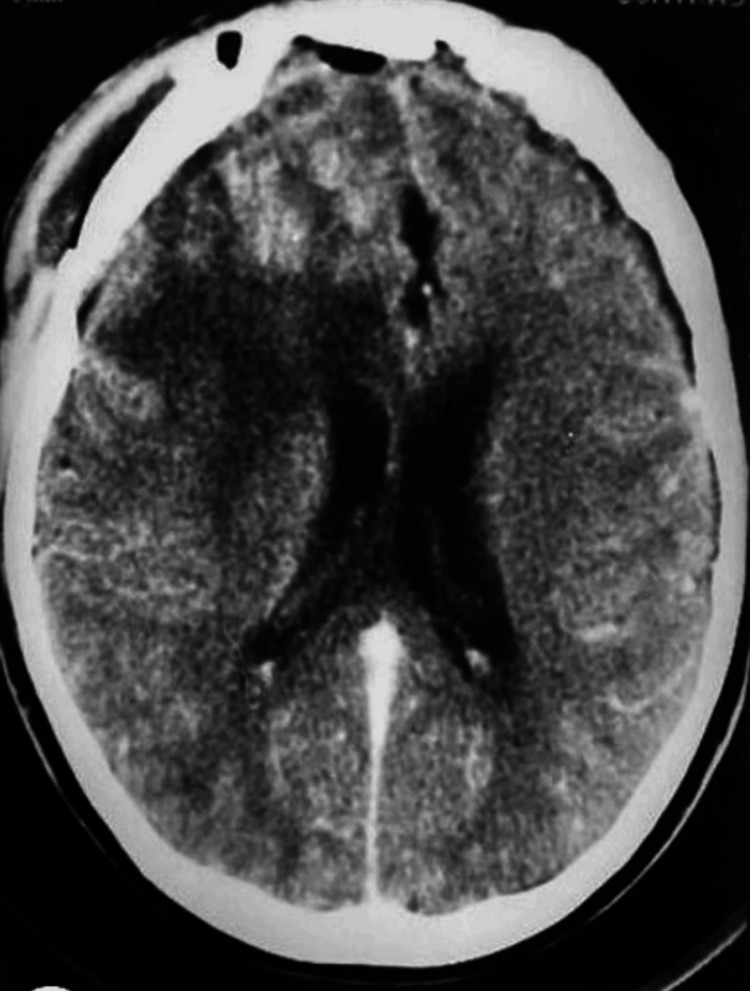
Postoperative brain CT scan after nine months showed no tumor
